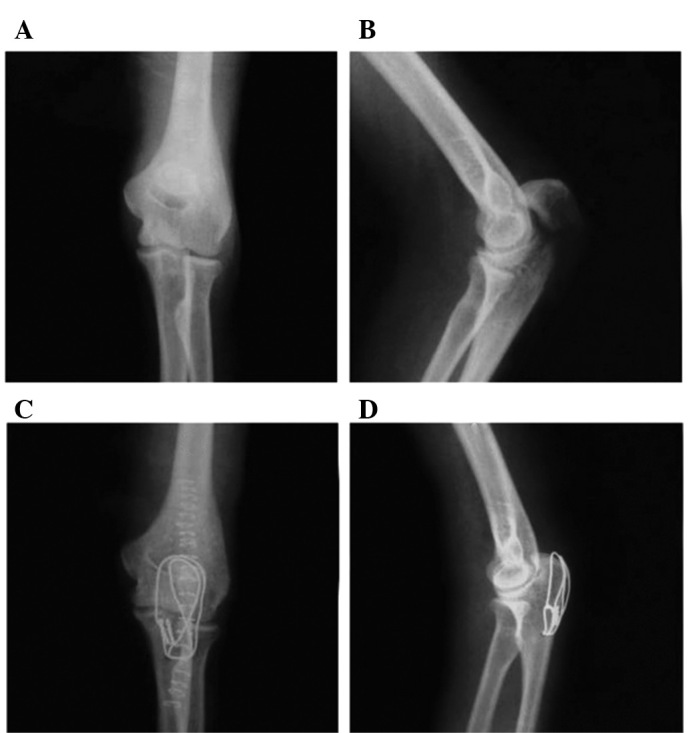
Representative X-ray films of olecranon fracture patients before and after treatment. (A) The positive X-ray film and (B) lateral X-ray film of olecranon fracture patients before treatment. (C) The positive X-ray film and (D) lateral X-ray film of olecranon fracture patients after treatment with figure of eight plus circular wiring, showing good positioning of the wires and an anatomical restoration of the fracture.
